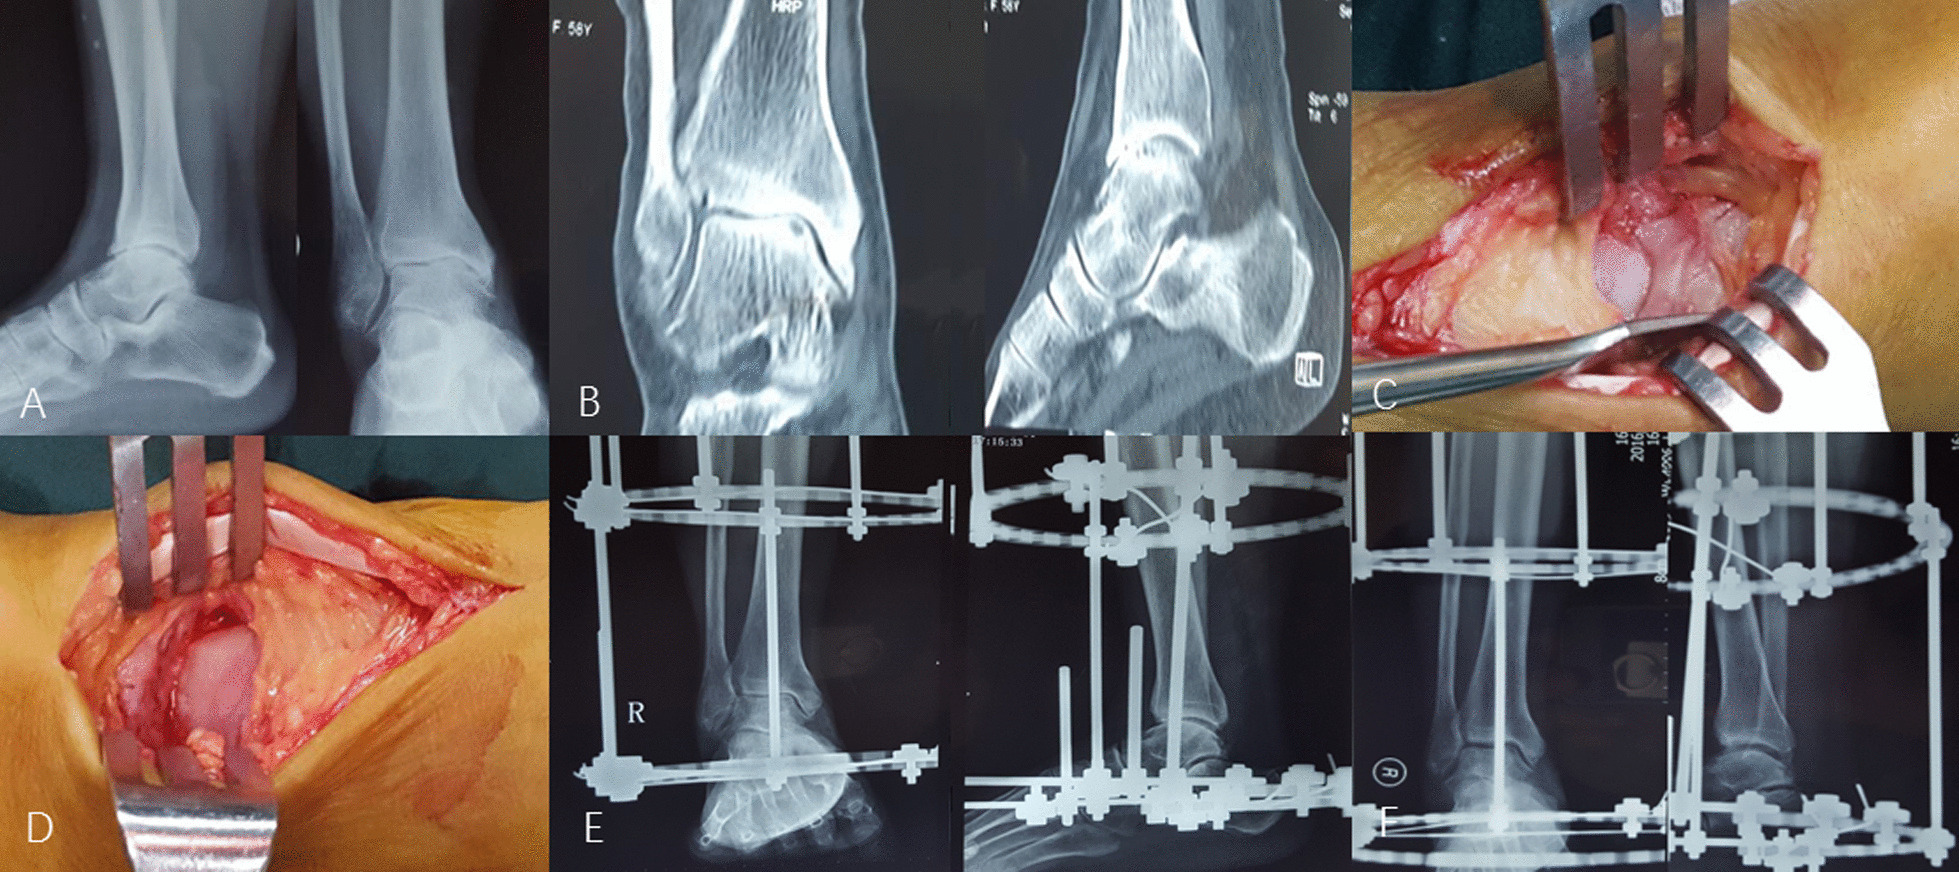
Depicts a female, 58 years, who had right post-traumatic varus ankle osteoarthritis classified as Takakura-Tanaka stage 3 and underwent ankle distraction arthroplasty and got a favorable outcome. The preoperative radiographs (A, lateral and anteroposterior view) and CT scans (B) narrow articular space and osteophytes, and the slight varus deformity. The osteophytes were seen and removed (C, D). seven days after operation, the ankle articular space was slightly widened (E). Radiographs taken at 1.5 months after operation showed marked improvement of ankle articular space, almost to normal (F)
